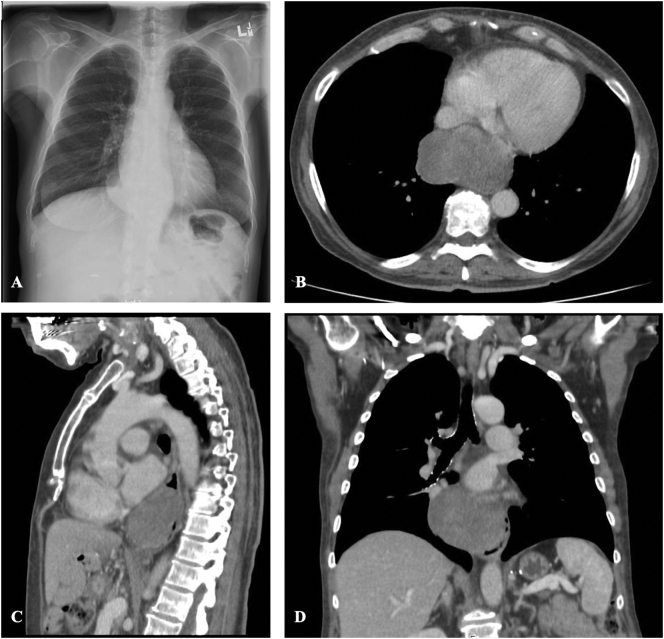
(A) Chest x-ray showing a large mediastinal mass. (B) Axial, (C) sagittal, and (D) coronal views of the chest computed tomography scan demonstrating the distal esophageal mass, 7.6 × 4.6 × 6.6 cm in size.
